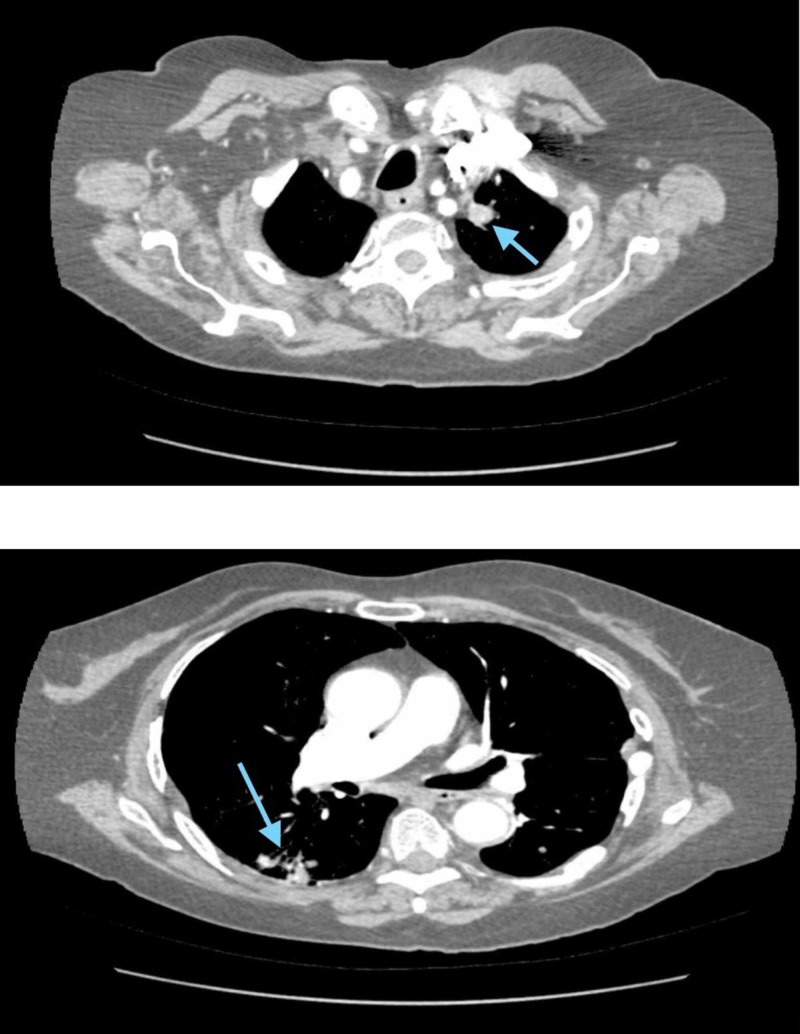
CT angiogram showing nodules in the left lung apex and posterior right lung.
